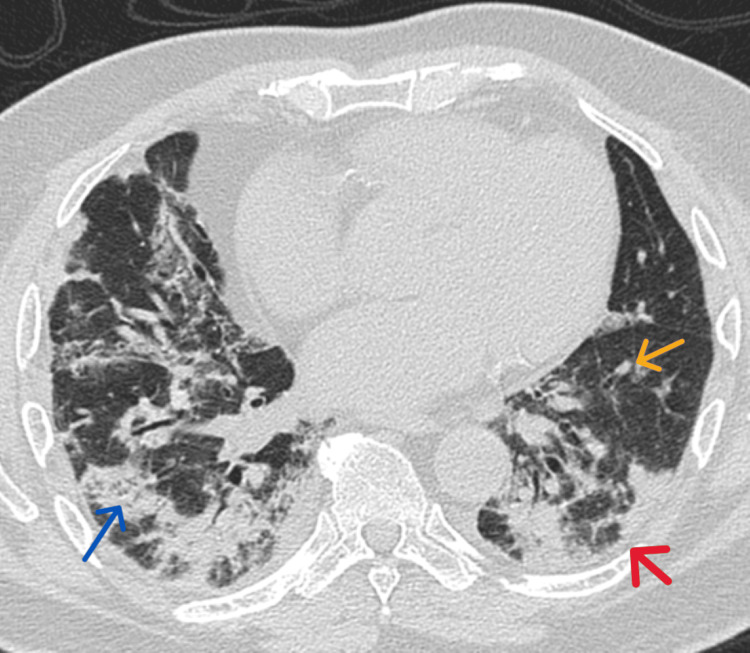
CT chest showing findings consistent with cryptogenic organizing pneumonia Blue arrow: consolidation, yellow arrow: nodules, pink arrow: perilobular pattern.
